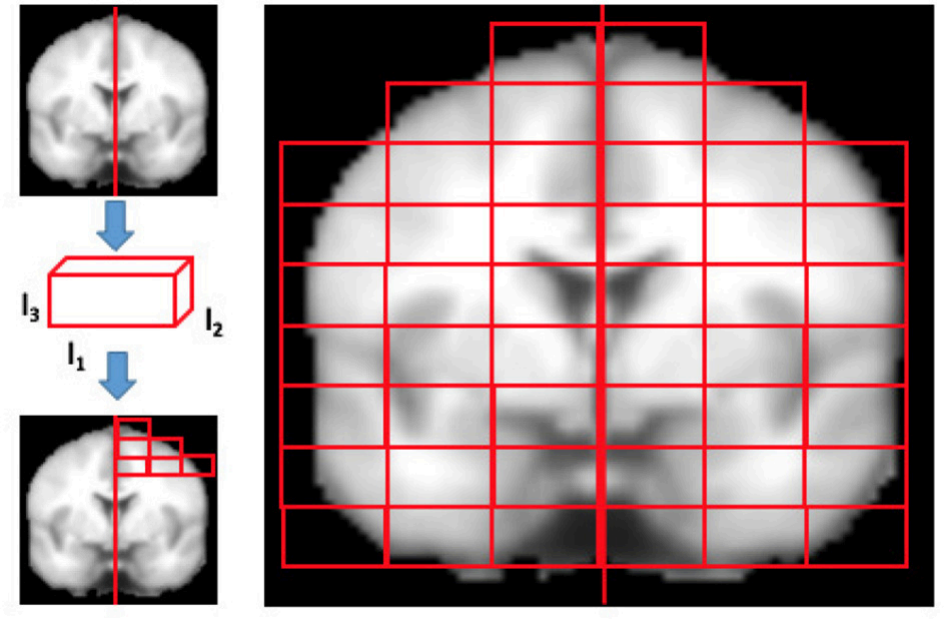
The figure qualitatively shows how MRI brain scans are segmented in rectangular patches of dimensions *l*_1_×*l*_2_×*l*_3_. Firstly, the brains normalized to MNI152 template are divided in left and right hemispheres using the medial longitudinal fissure, then the patch dimensions are set and finally the brain is segmented. Only patches overlapping the brain for at least the 10% of their content are kept, others are discarded.
